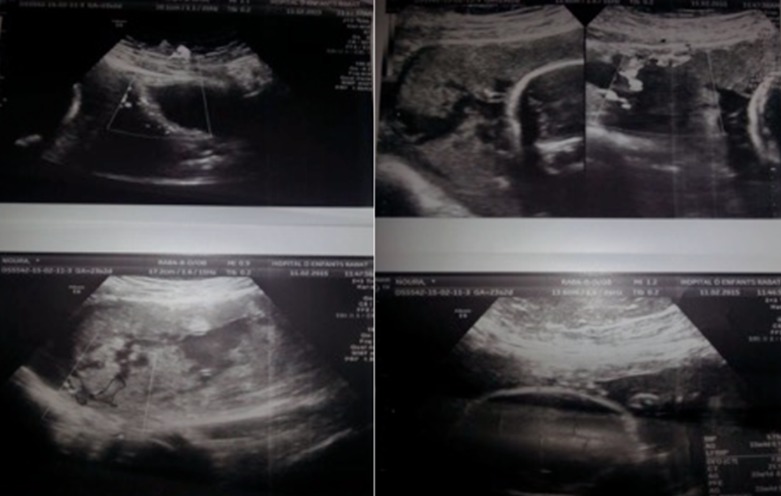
Doppler ultrasound showing vascularization crossing most of the thickness of the myometrium: placenta accreta
